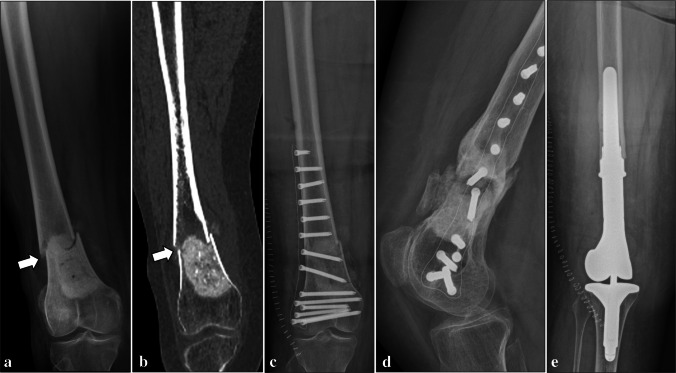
Post-operative bone fracture in a 53-year-old woman. Bone fracture (arrows) occurred 2 months after curettage of an ACT of the distal femur, as shown on radiograph (a) and CT (b). Treatment consisted of internal fixation using screws and plate (c). However, non-union occurred (d), and wide resection and reconstruction with knee megaprosthesis were finally needed (e)
